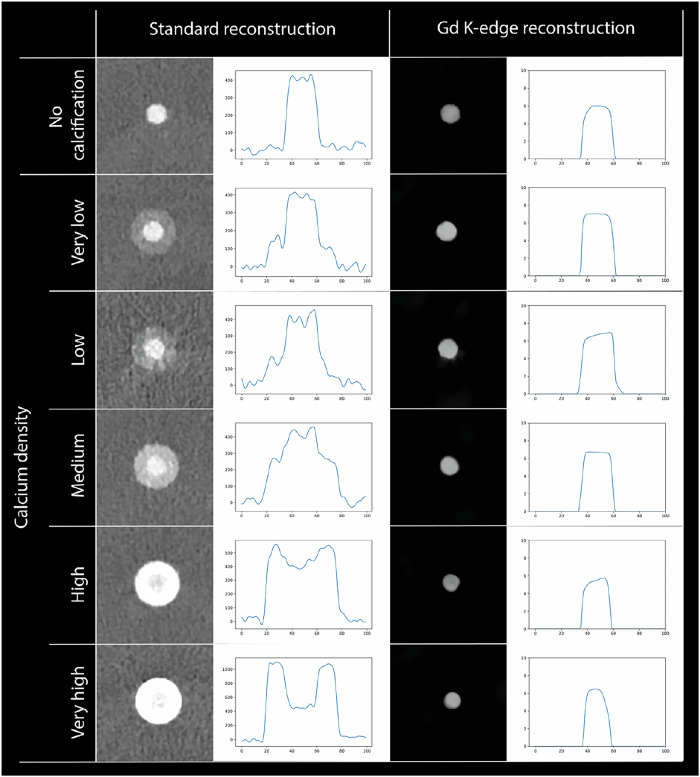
Standard conventional and color Gd K-edge images in combination with a Gd-based contrast agent for a noncalcified plaque and all five calcification densities. For both reconstructions, the image data (left) and a profile plot through the center of the lumen (right) are shown. For both profile plots, the x-axis shows the distance, while the y-axis shows the CT number (HU) or the Gd-concentration (mg/mL), for the conventional and K-edge reconstruction, respectively. For the CT image data, a window-width/window-level setting of 750/90 HU was used. For the color Gd K-edge image data, the values represent a minimum of 0 until a maximum of 10 mg/mL of Gd
